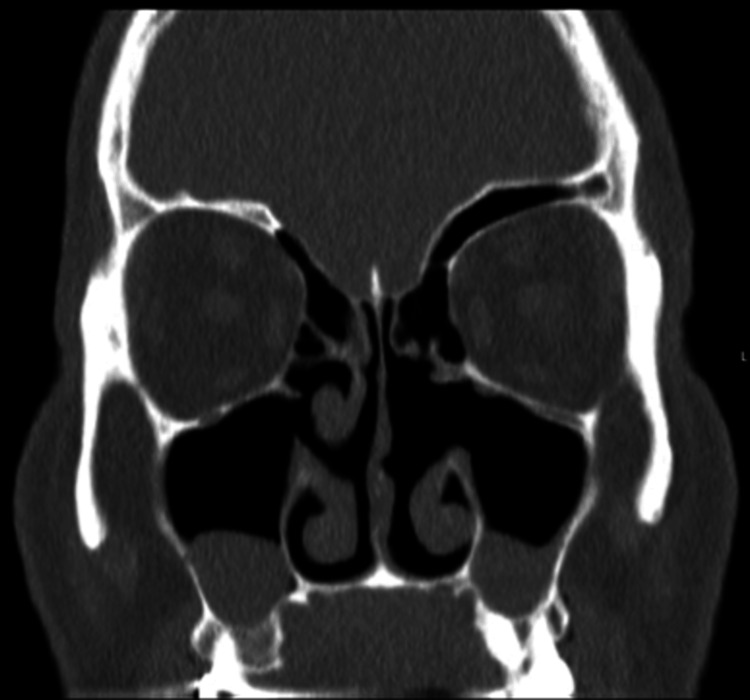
Post-operative computed tomography of the paranasal sinuses showing resolution of the primary disease
